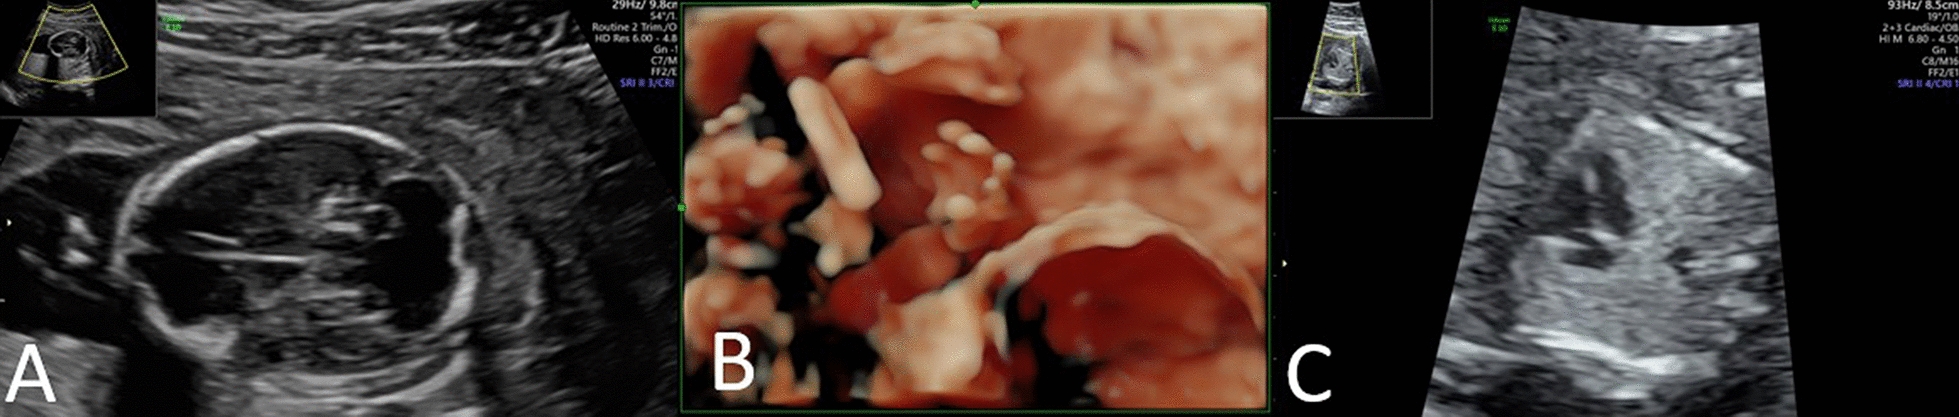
Ultrasound anomaly scan A Midline defect cerebral (compatible with holoprosencephaly) B Polydactyly and C Congenital cardiac defect (suspect for ventricular septal defect)
